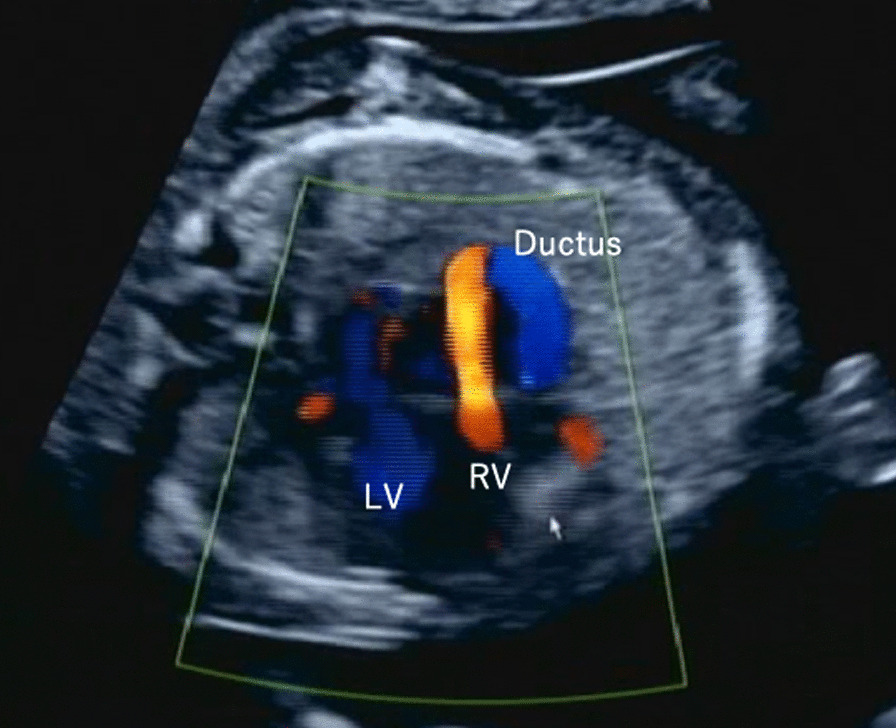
Fetal ultrasound images; 13 weeks and 3 days of gestation in the second pregnancy. A close examination of the heart revealed cardiac enlargement with a cardiothoracic area ratio (CTAR) > 35% and no pulmonary artery valve. The ductus arteriosus was retrograde. LV, left ventricle; RV, right ventricle
